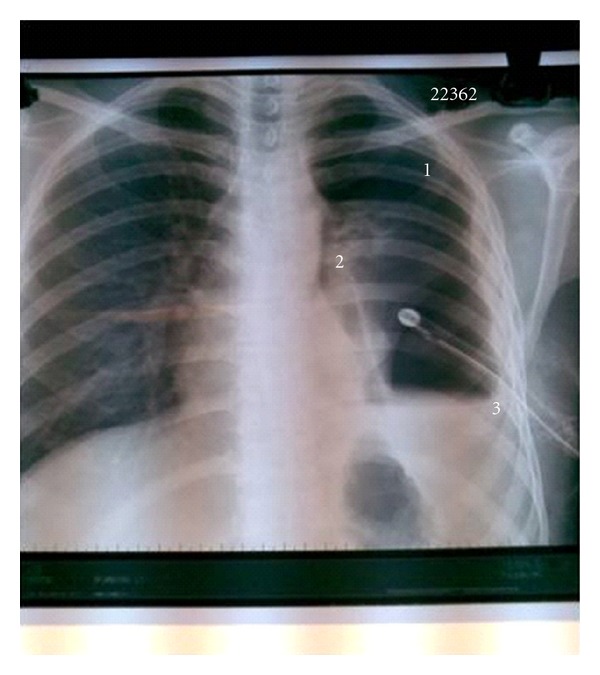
Chest roentgenogram showing massive left pneumothorax (1) with collapsed left lung (2). Intercostal drain is seen in situ with residual pyothorax (3).
